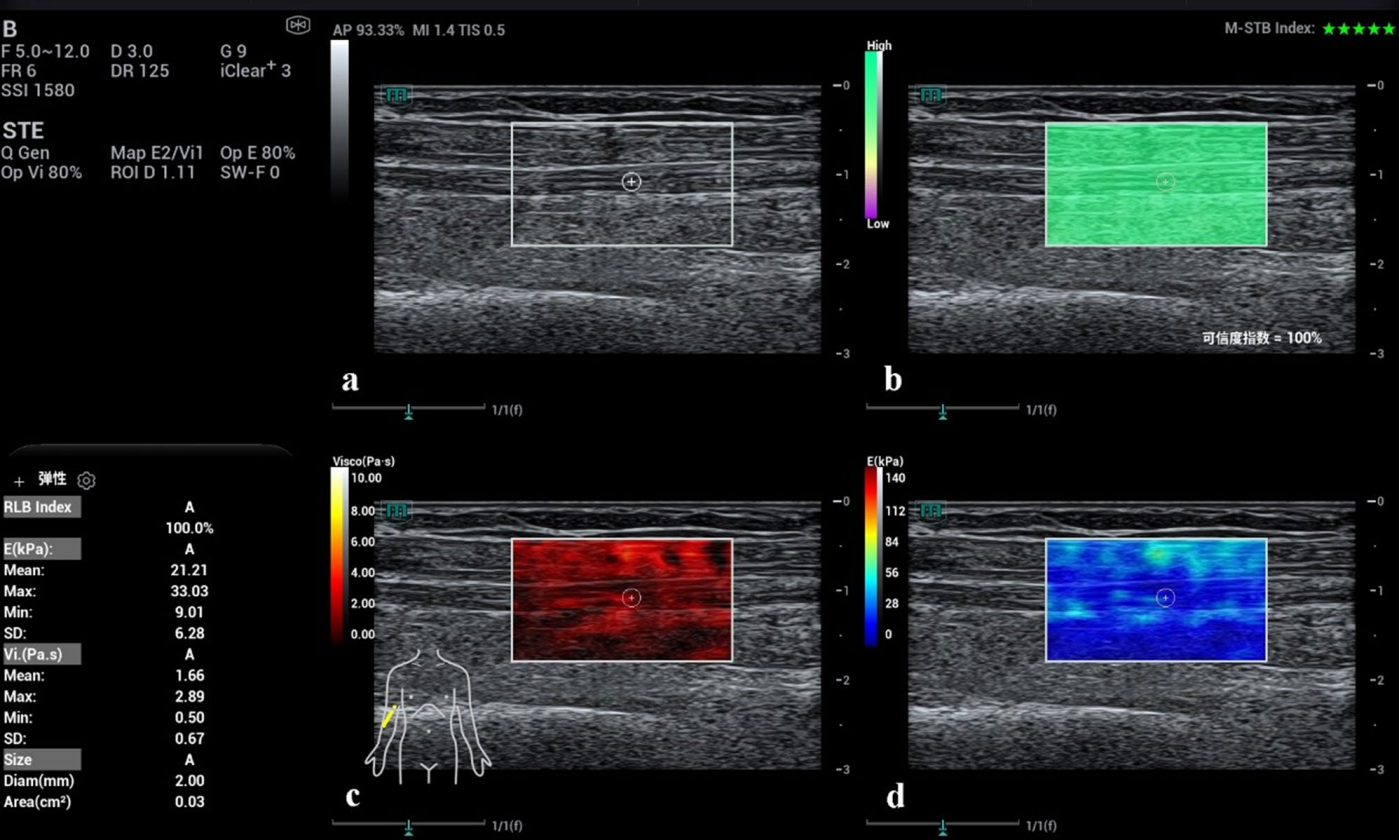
Representative STVi measurement of the right MN3 in a healthy subject. (a) Grayscale ultrasound image; (b) Reliability (RLB) map indicating high data quality (green); (c) Viscosity map showing localized distribution (range 0–10 Pa·s); (d) SWE map displaying stiffness distribution (range 0–140 kPa). Mean viscosity and stiffness values are displayed in the lower-left corner
